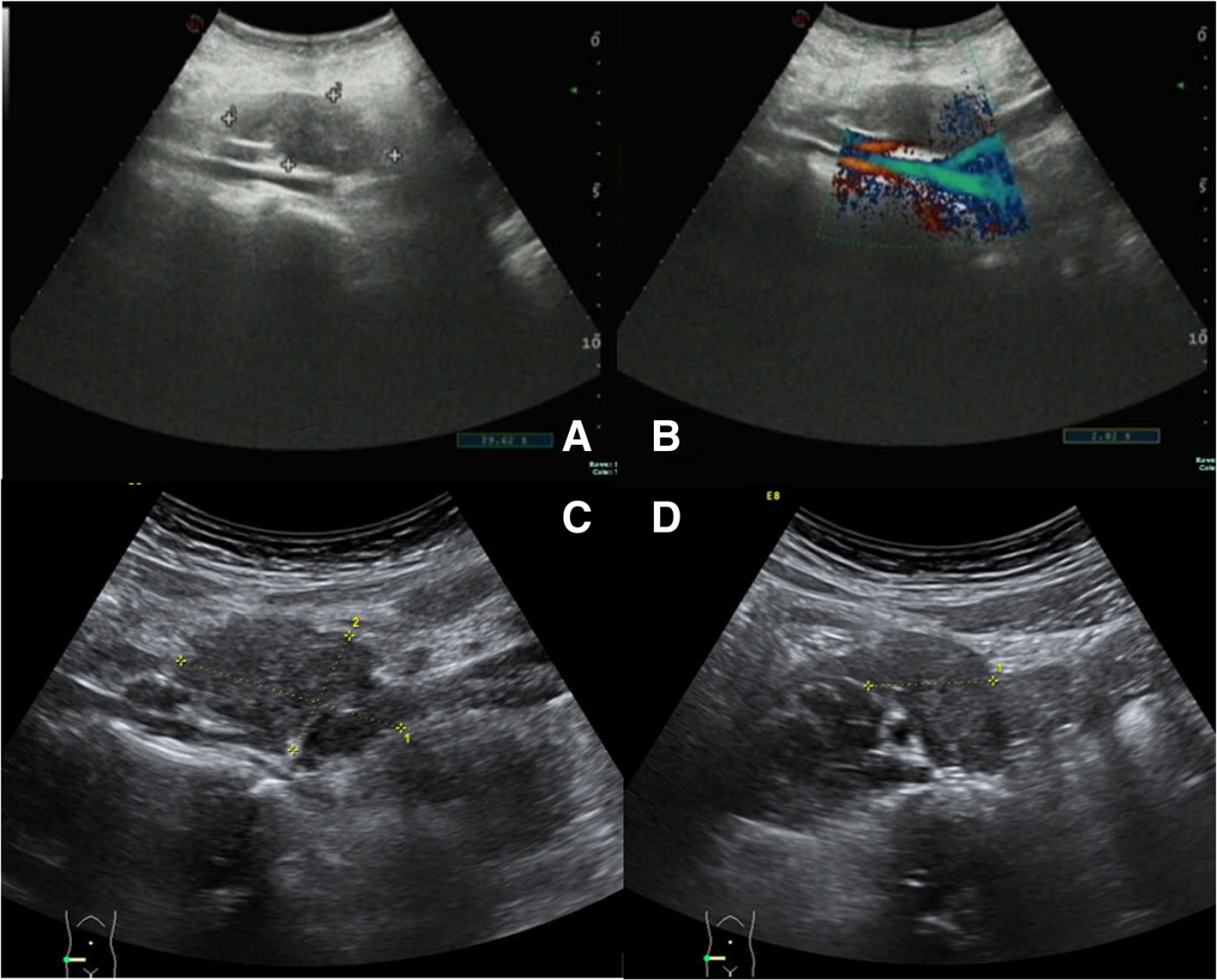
Preoperative imaging. a and b Color Doppler ultrasound (double kidneys, ureters, bladder); c and d: Transvaginal color Doppler ultrasonography
